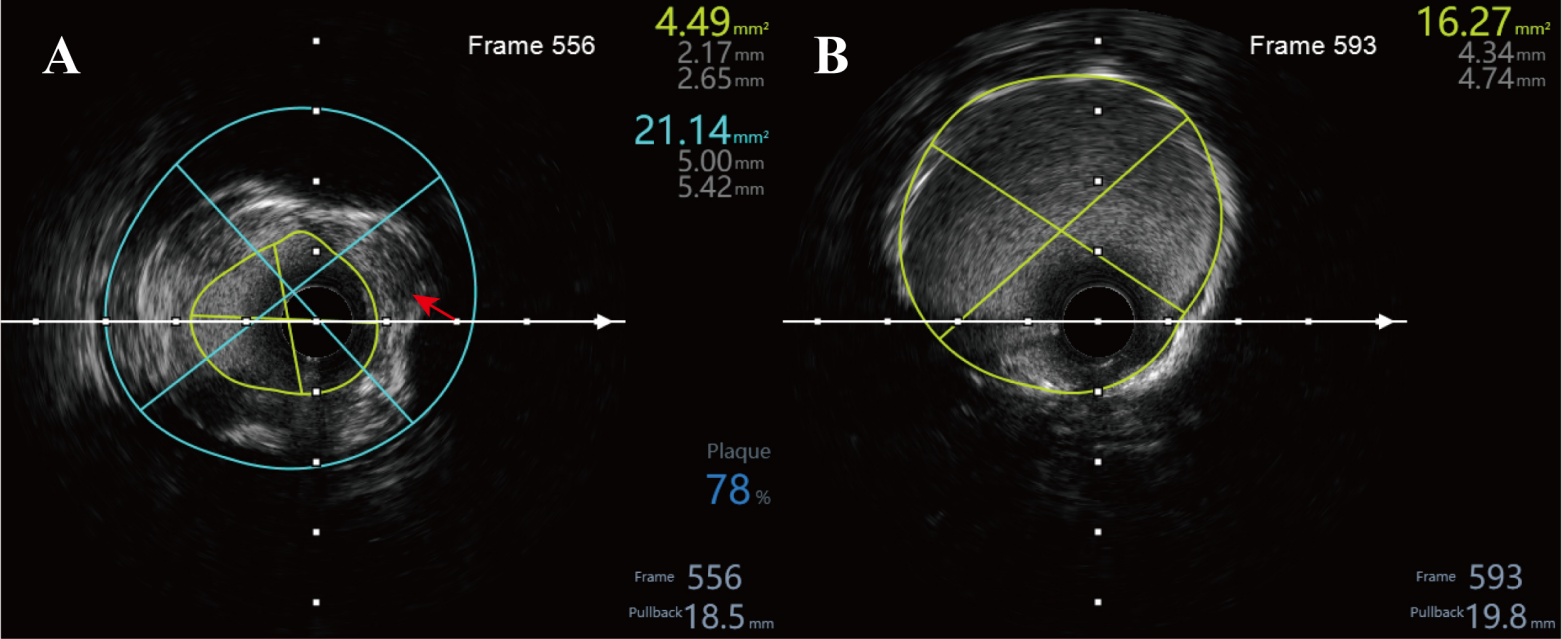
Intravascular ultrasound (IVUS) assessment. (A) After balloon
